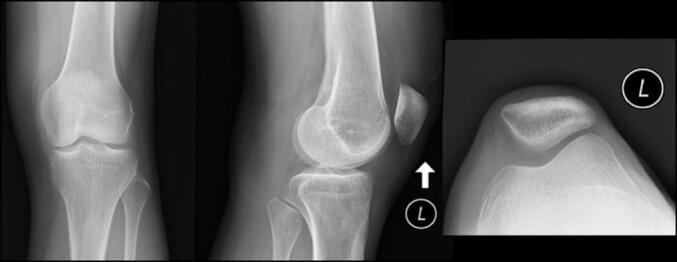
The plain radiograph of the left knee joint.
